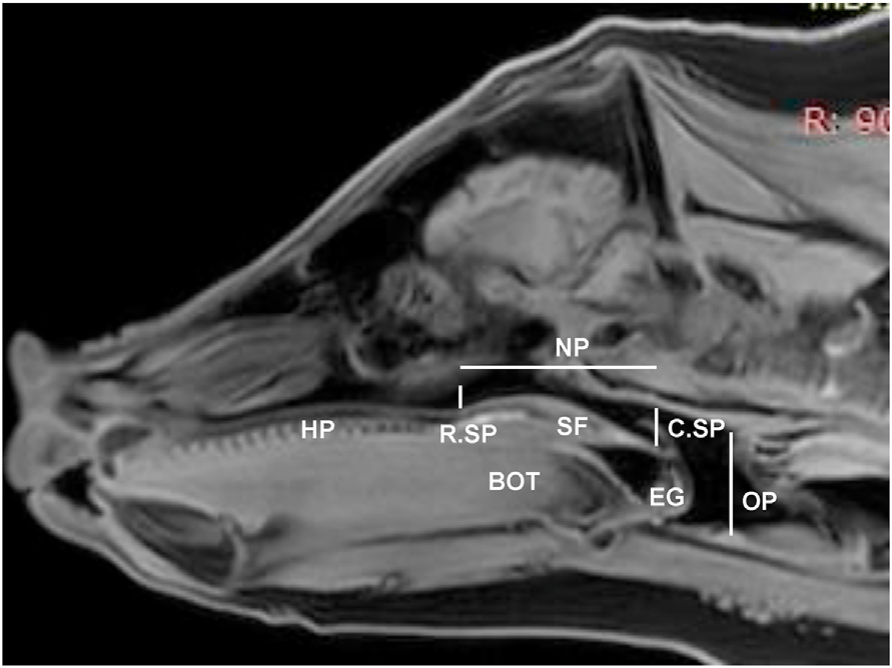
VISTA MRI sagittal slice by RadiAnt DICOM Viewer to measure dimensions of nasal and oral pharyngeal airway spaces. HP: Hard palate; SF: Soft palate; BOT: Base of the tongue; EG: Epiglottis. Two vertical lines indicate the rostral and caudal ends of soft palate (R.SP and C.SP). Horizontal and vertical dotted lines indicate the locations of nasal and oral pharyngeal airway spaces (NP and OP), respectively.
